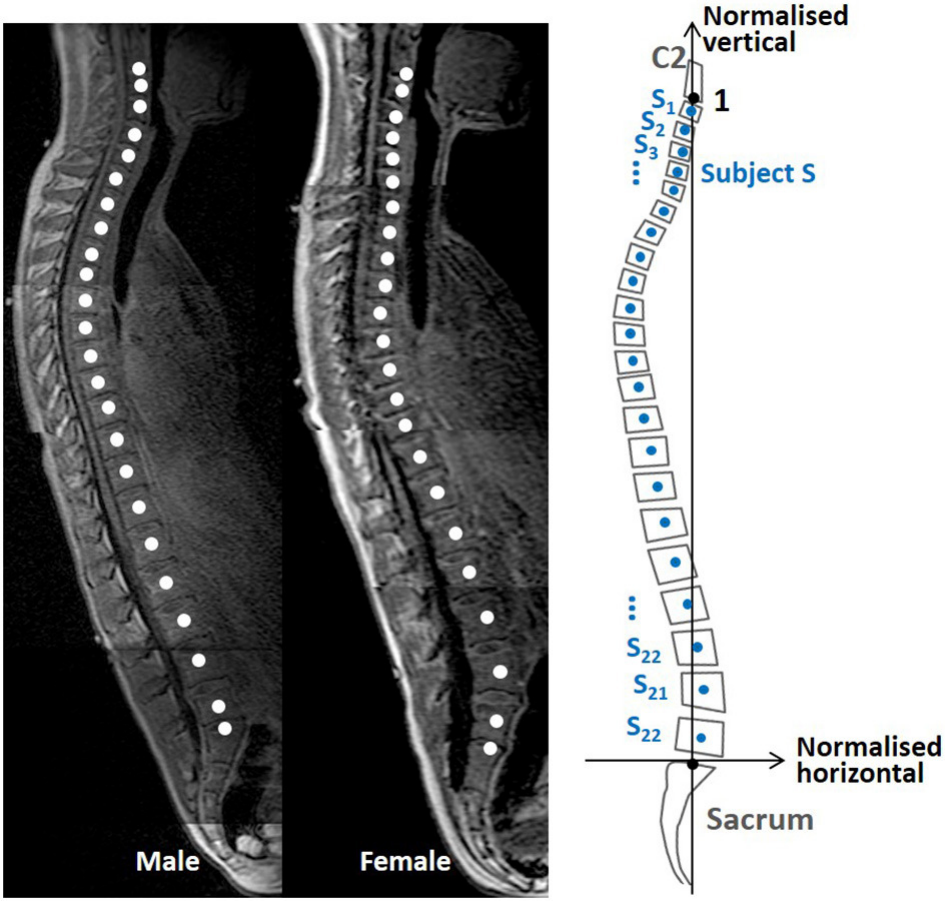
Magnetic resonance imaging data spinal alignment and transfer to a normalized coordinate system.
